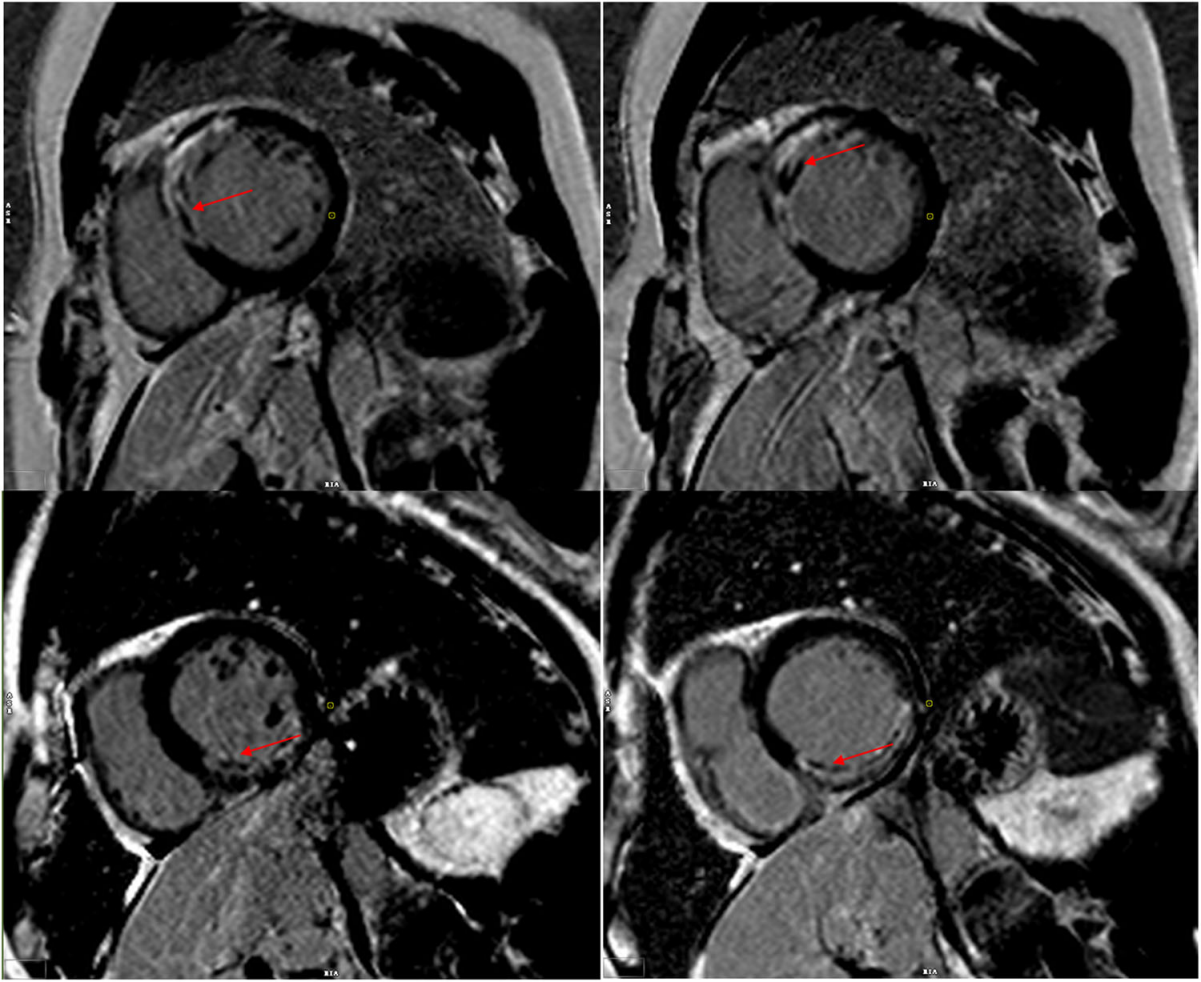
The cardiac magnetic resonance imaging reveals regions of diminished signal intensity in the enhancement, indicative of coronary microcirculatory dysfunction.
